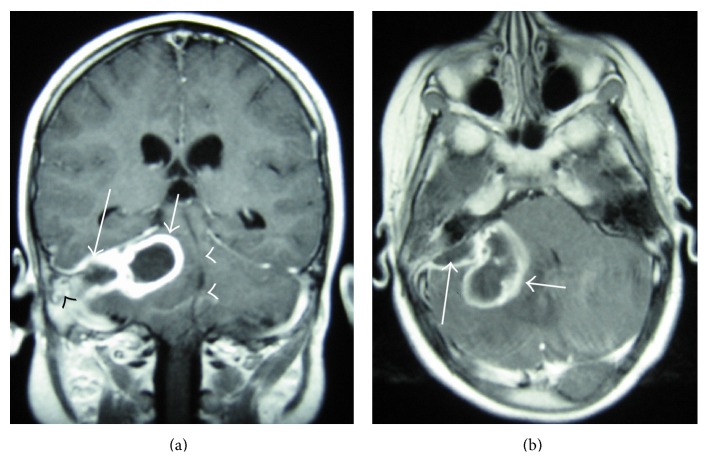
Coronal (a) and axial (b) MRI sections with contrast on admission, showing a large cerebellar abscess on the right hemisphere (short arrows) coexisting with an epidural abscess of the posterior fossa (long arrows) and inflammatory tissue within the mastoid cavity ((a), black arrowhead). The abscesses exert mass effect against the middle-line structures, causing their displacement to the left ((a), white arrowheads).
